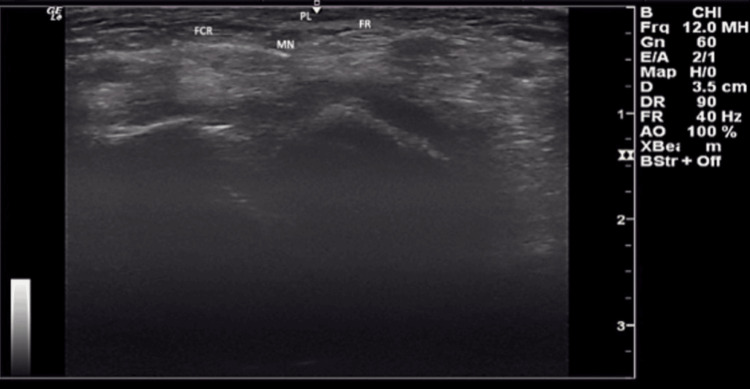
Confirmed Presence of Tendon by Ultrasound Palmaris longus (PL), as labeled in the figure, is identified in the anterior compartment of the forearm, superficial to the flexor retinaculum (FR). Relevant structures, including the flexor carpi radialis (FCR), visualized laterally, and the median nerve (MN), visualized posteriorly, are also shown.
